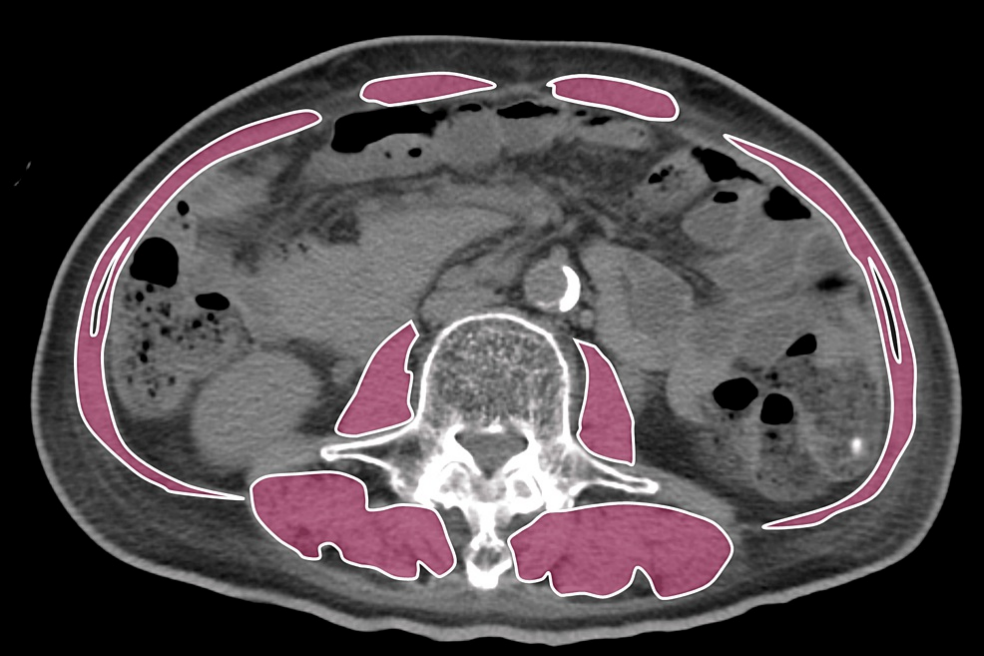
Representative abdominal CT images of sarcopenia Demonstrable axial CT images at the third lumbar vertebra region with highlighted skeletal muscles. The image was obtained from LightSpeed VCT Vision (GE Healthcare, Princeton, NJ, USA) and analyzed with Ziostation2 (Ziosoft, Tokyo, Japan). CT, computed tomography
